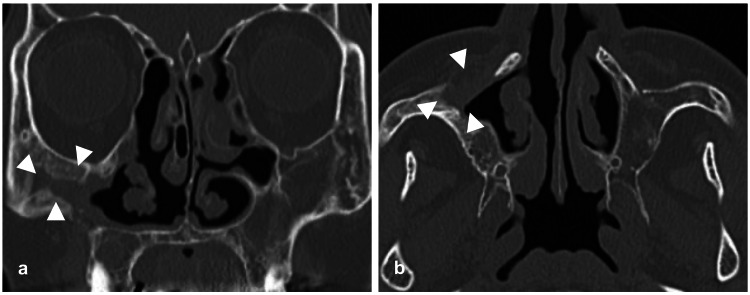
Postoperative CT images The cyst remained open and spontaneously contracted. White arrowheads indicate the cyst. (a) shows a coronal slice and (b) shows an axial slice.
